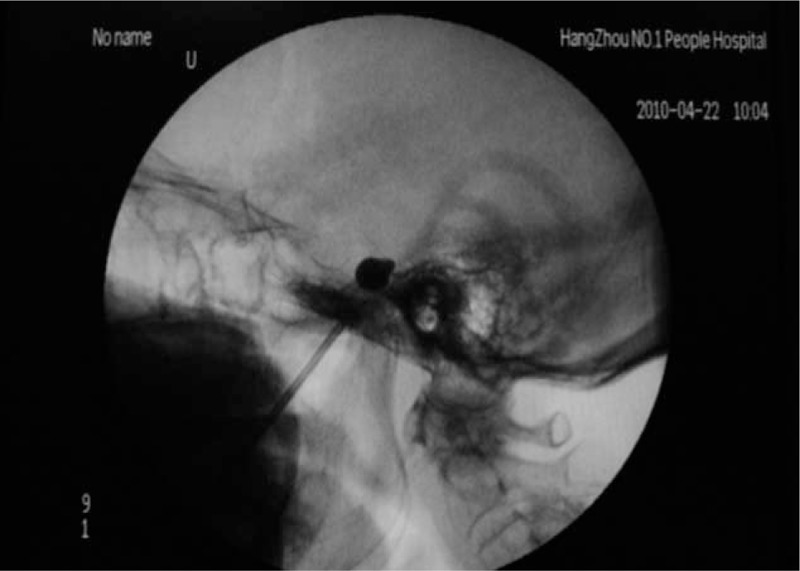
The filled balloon exhibited a pear-like shape as shown by lateral X-ray fluoroscopy.
